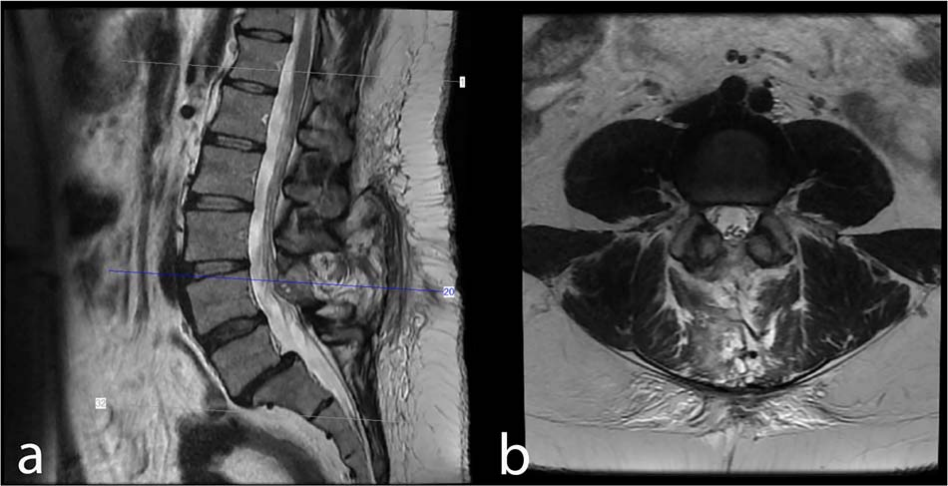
Forty-three month postoperative (a) midsagittal and (b) axial MRI images show no residual/recurrent intradural lesion.
